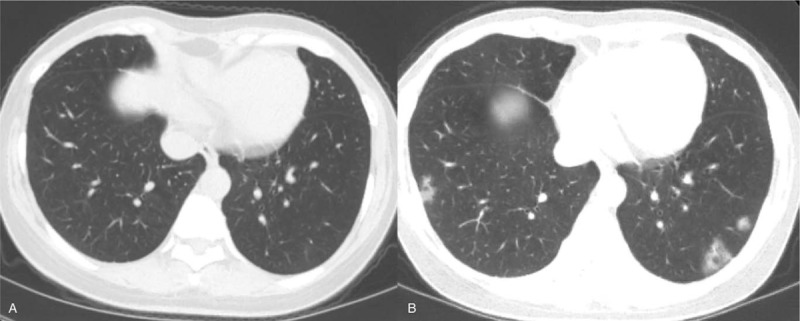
In a 39-year-old asymptomatic female with coronavirus disease 2019 by second-generation infection, no abnormal findings have been found on initial computed tomography scan after admission (A) but positive nucleic acid test on admission. On the follow-up computed tomography on day 8 after admission, focal patchy ground glass opacities appear in the lower lobes of both lungs (B).
